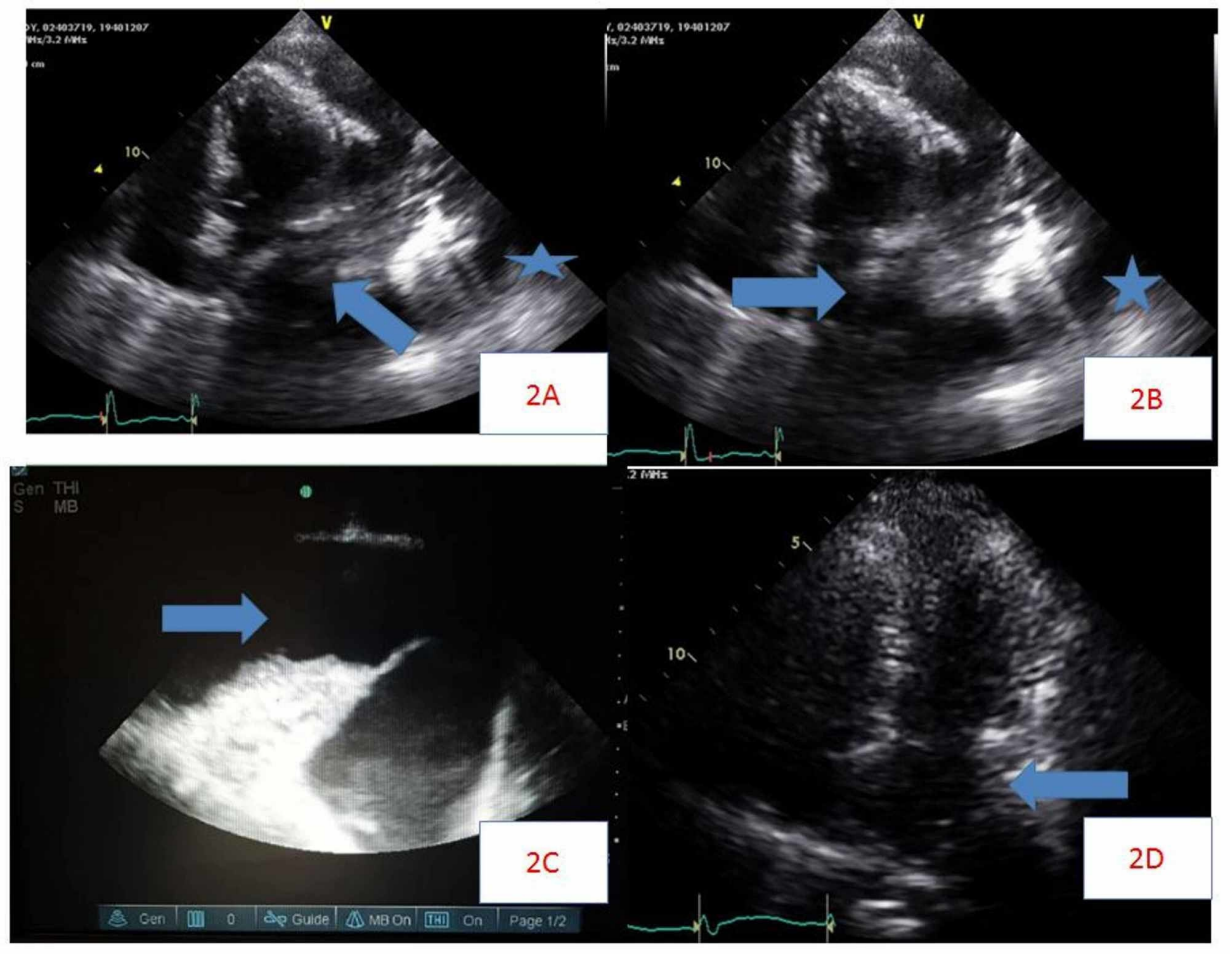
Echocardiogram findings Echocardiogram showing diastolic (A) and systolic (B) collapse of left atrium (arrow) due to pleural effusion (star) causing cardiac tamponade. Thoracic ultrasound (C) showing large left pleural effusion (arrow). Echocardiogram done after thoracentesis (D), showing no diastolic collapse of the left atrium (arrow), with resolution of cardiac tamponade
